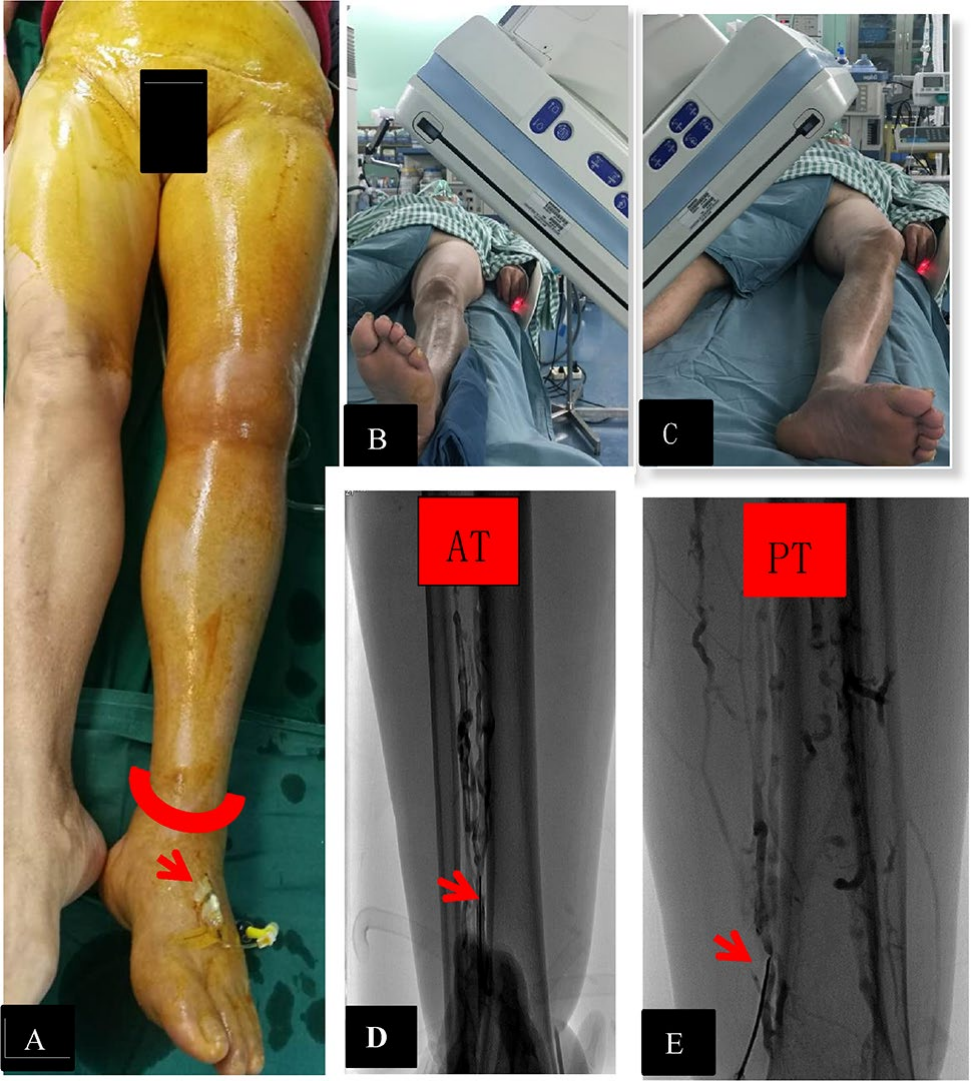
Overview of the calf deep vein puncture procedure. (A) The entirety of the affected limb and the contralateral inguinal region were sterilized, and an indwelling needle was inserted into the dorsal vein of the foot with the elastic bandage on the ankle (Arrow and Semicircle). (B) The position of the DSA guidance for anterior tibial vein and peroneal vein puncture. (C). The position of the DSA guidance for posterior tibial vein puncture. (D, E) Puncture of the anterior and posterior veins was performed under real-time X-ray guidance using an appropriate contrast agent.
